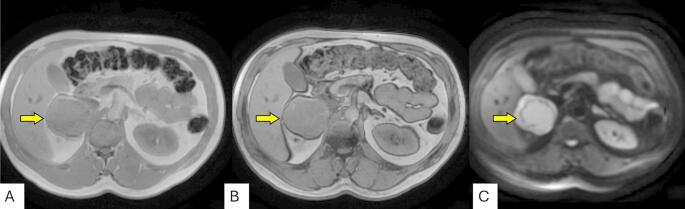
Axial MRI of the abdomen showing a right adrenal mass (yellow arrows) across in-phase (A), out-of-phase (B), and diffusion-weighted imaging (DWI) sequences (C). A, B The lesion demonstrates no significant signal drop between in-phase and out-of-phase T1-weighted images, suggesting absence of intracellular lipid, which helps exclude lipid-rich adenoma. C Diffusion-weighted image shows restricted diffusion within the solid components of the mass, a finding often associated with hypercellularity in malignant pheochromocytoma
